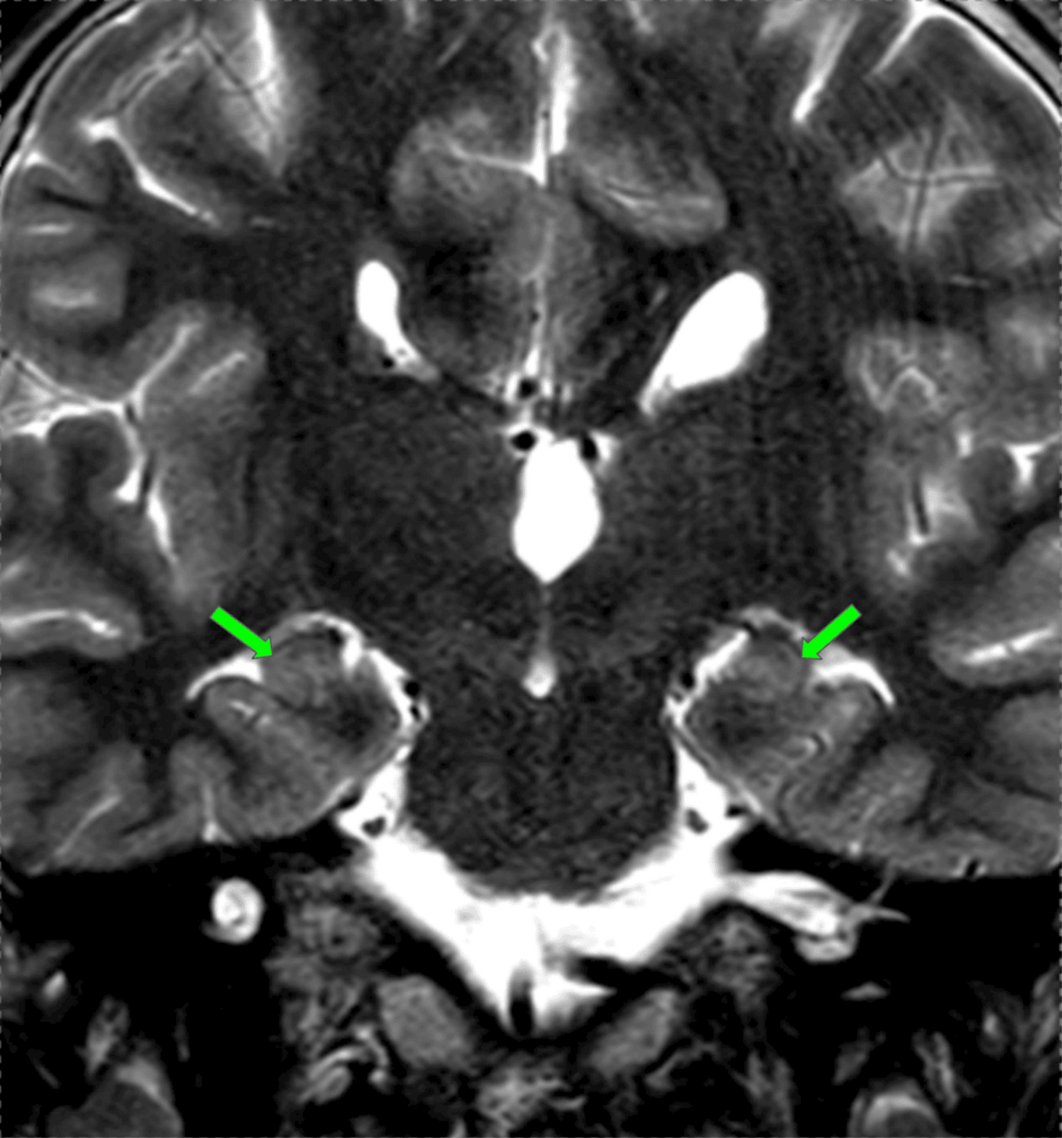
Coronal T2-weighted MRI of the brain without contrast demonstrates dysplasia, malrotation, and an ovoid shape of the hippocampi bilaterally (green arrows) T2 - transverse relaxation time
